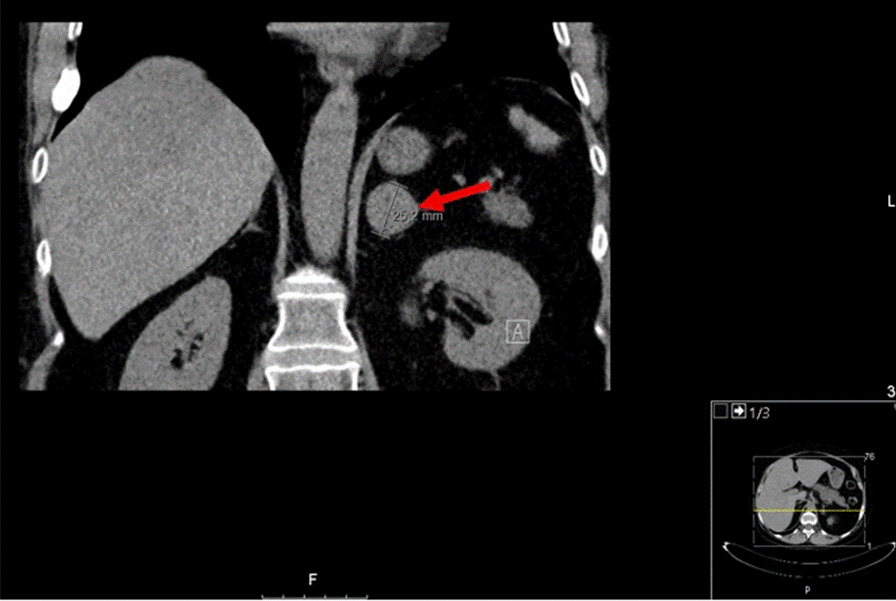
Computed tomography (CT) scan showing a 2.5 mm adenoma of the left adrenal gland (red arrow)
